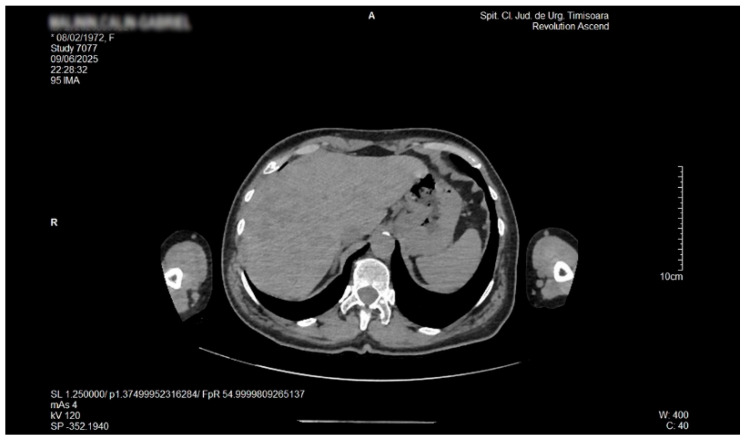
Native axial abdominal CT. Axial non-contrast CT image at a slightly lower hepatic level showing persistent multifocal hypodense lesions, highlighting the diffuse distribution of parenchymal lipid deposition characteristic of the focal disseminated steatosis variant.
